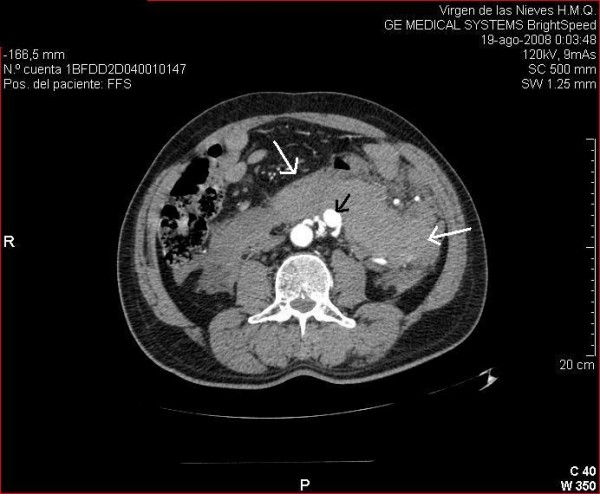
Retroperitoneal haematoma. CT image demonstrating a huge retroperitoneal hematoma (white arrows) and the IMA aneurysm (black arrow).
